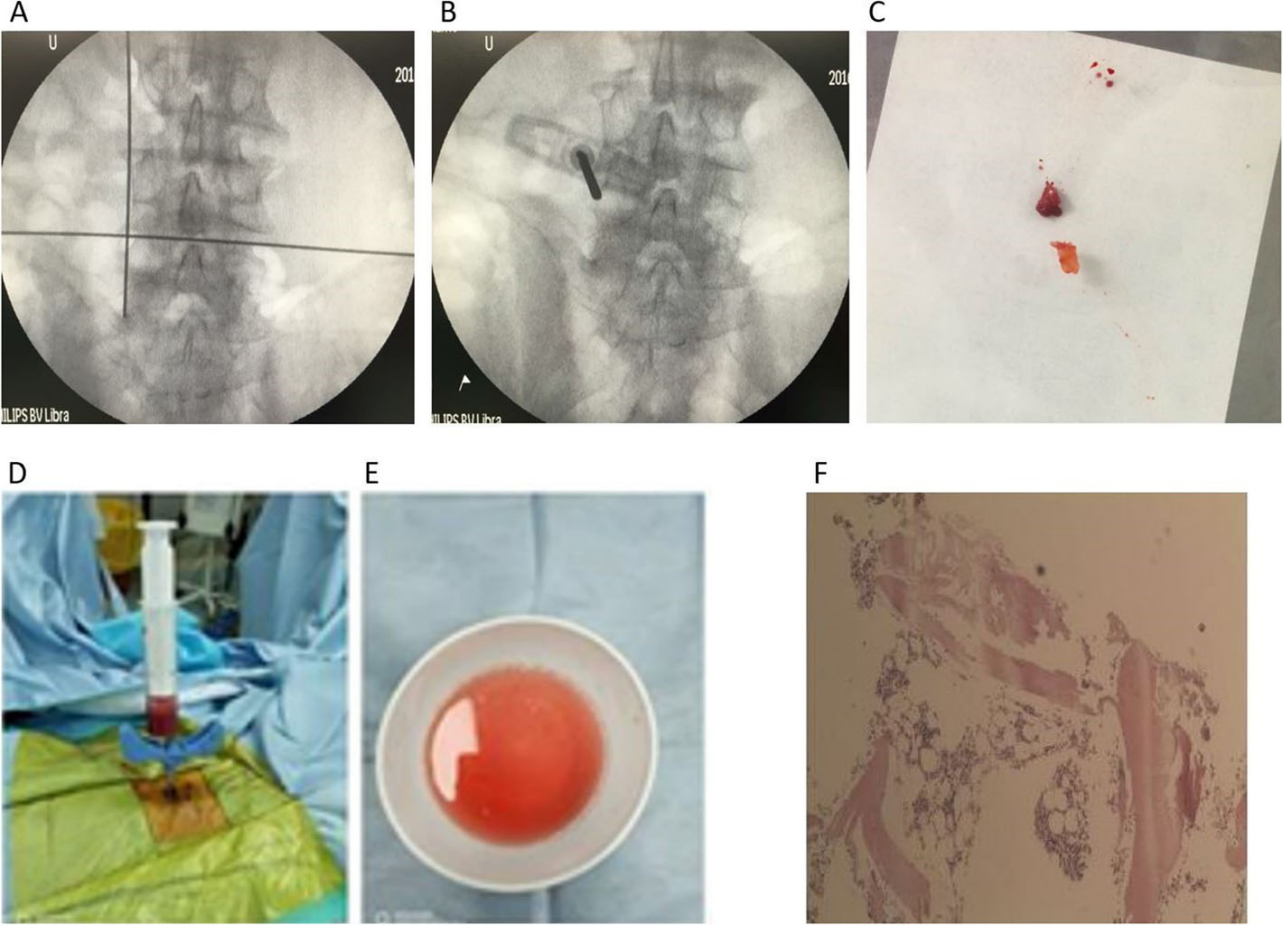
Pictures during the operation and pathological report of L5 bony tissue biopsy. Notes: (a and b) X-ray pictures of fluoroscopy during operation, L5 vertebrae was punctured from left pedicle by needle. (c) bony tissue was obtained from L5. (d and e) Sterile saline solution was irrigated through needle into L5 by syringe and collected for aerobic and anaerobic bacteria culture and drug susceptibility test. (f) Pathological report showed diffused distribution of plenty of lymphocytes without the gathering of epithelioid cell granulomas, granular necrosis and Langerhans giant cells
